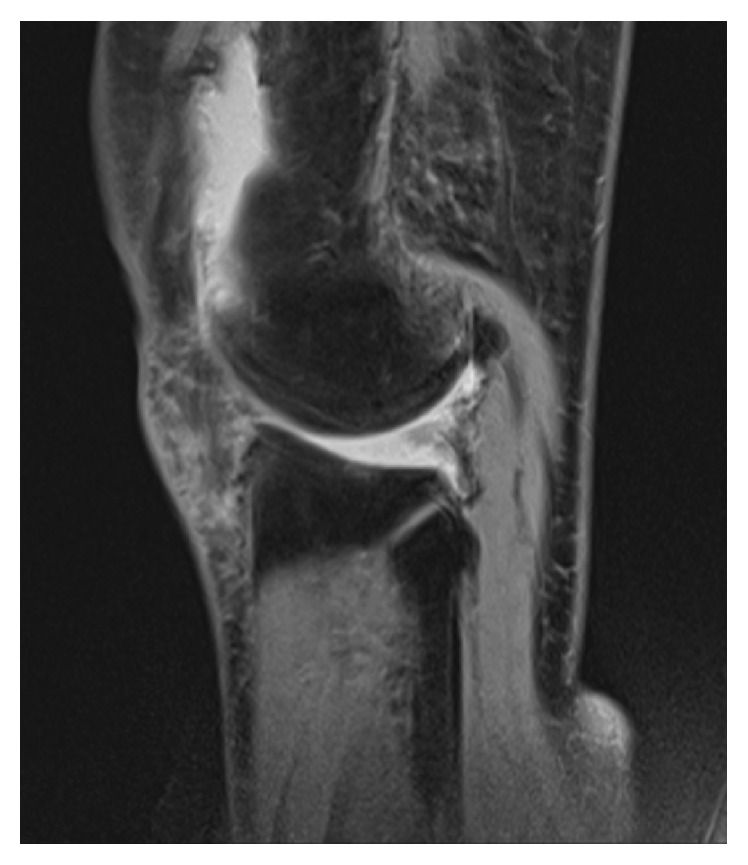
MRI of Case 2 (T2 weighted image). The middle and posterior portions of the lateral meniscus are torn.
